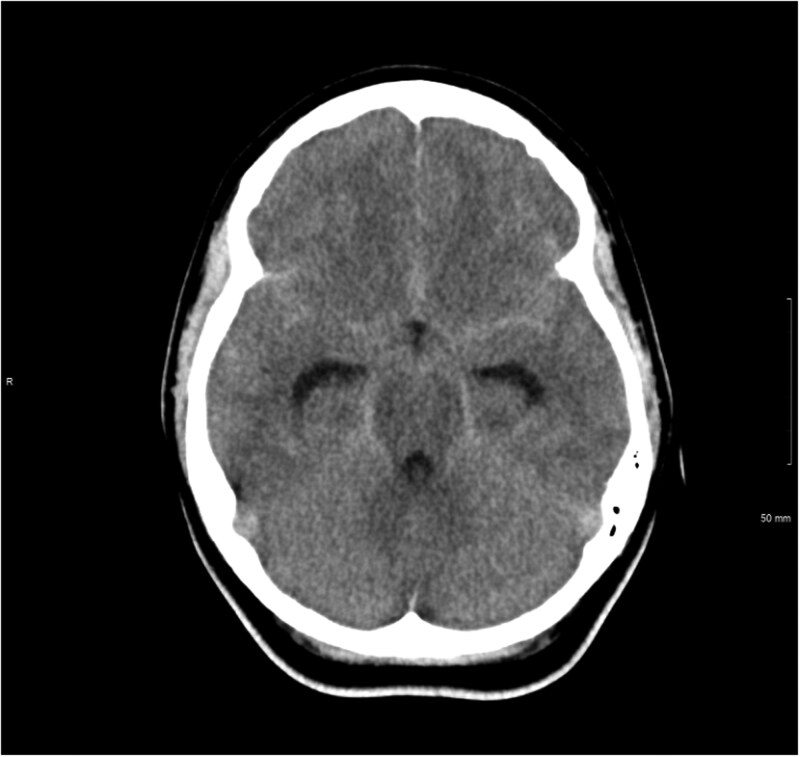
CT head at presentation demonstrating diffuse SAH with dilatation of the temporal horns indicating acute hydrocephalus.
